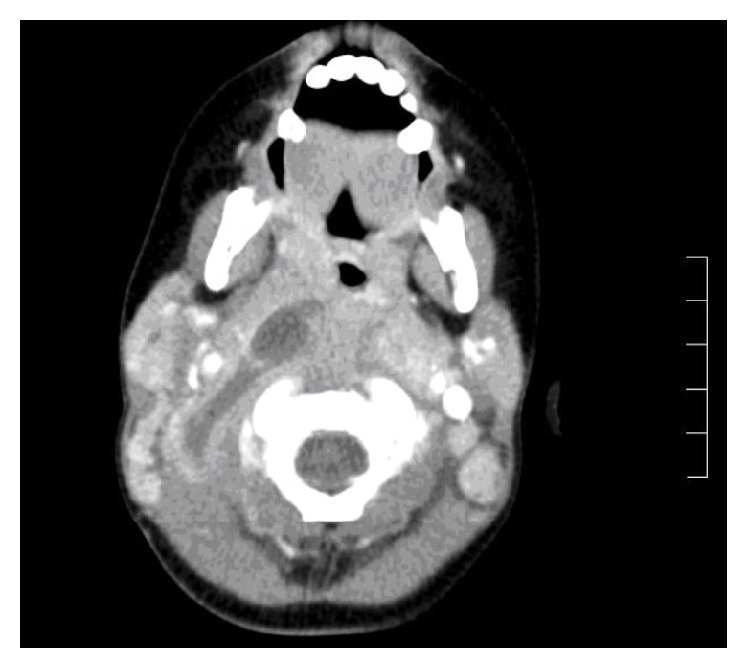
CT shows a hypodense area in the right parapharyngeal region, surrounded by an area of soft tissue thickening. There is minimal ring enhancement of the lesion.
